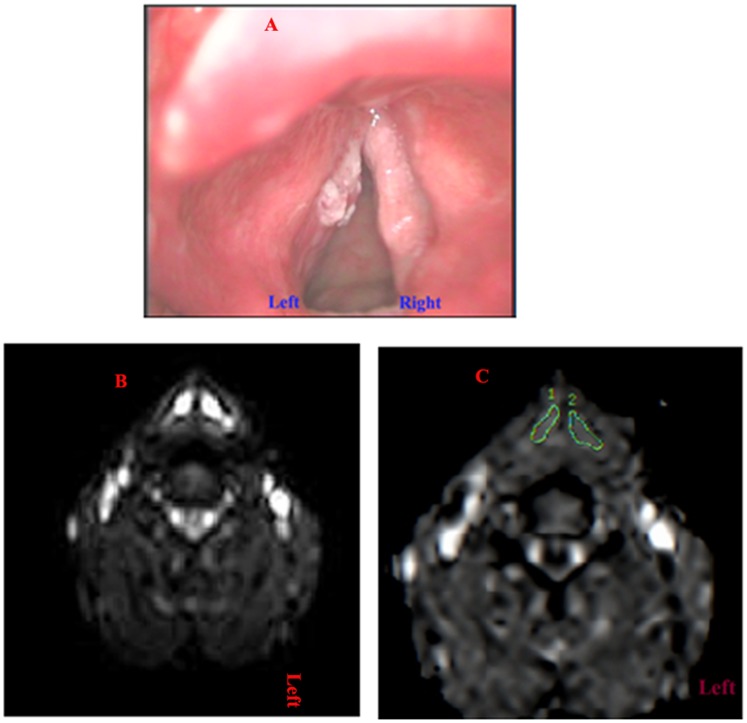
A patient with pathologically proven laryngeal carcinoma in the left vocal cord and mild dysplasia in the right vocal cord. A: Laryngostroboscopy showed a 5 × 6-mm mass in the left vocal cord and rough mucosa in the anterior right vocal cord suspected to be bilateral laryngeal carcinoma in the vocal cords. B: DWI-suggested lesions were identified in the bilateral vocal cords (b = 1000 s/mm^2^). C: Consequently, the corresponding ADC map reveals hypointense of lesions in the left vocal cord. (ADC = 1.26×10^−3^ mm^2^/s), and hyperintense of lesions in the right vocal cord(ADC = 1.67×10^−3^ mm^2^/s).
